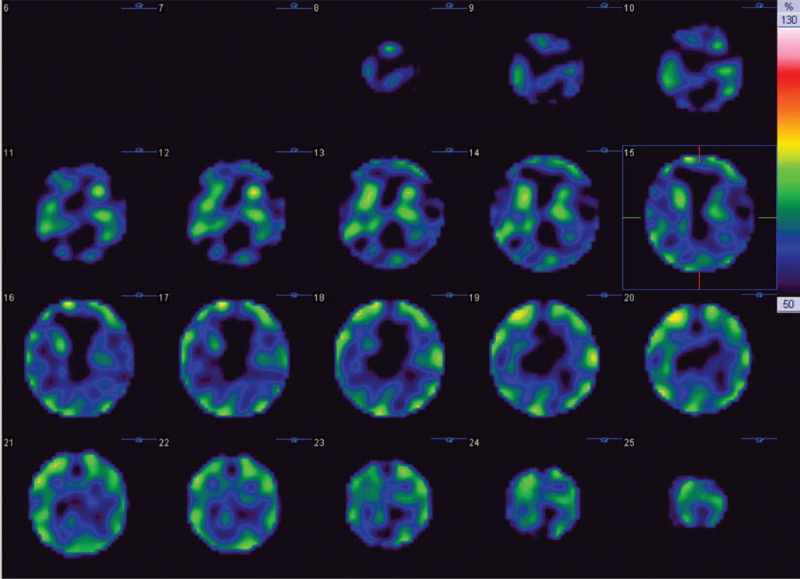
The axial technetium-99m-labeled tropanes as dopamine transporter imaging agents brain single-photon emission computed tomography scan of the patient. The images show marked reduction in the expression of dopamine transporter of the putamen and caudate nuclei of both sides. The qualitative visual scale of striatal uptake was 4. Tc-99m TRODAT-1 = technetium-99m-labeled tropanes as dopamine transporter imaging agents, SPECT = single-photon emission computed tomography.
